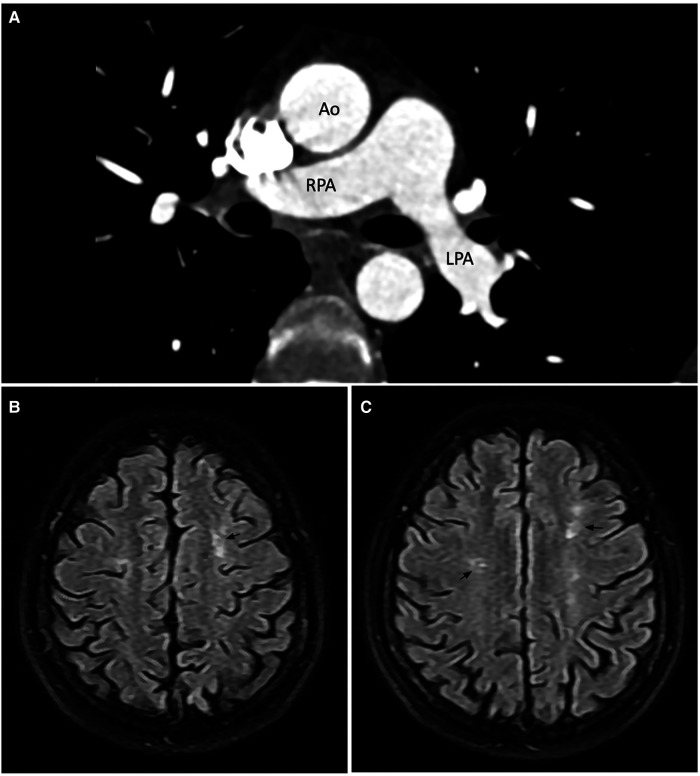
Computed tomography pulmonary angiogram (A) and brain magnetic resonance imaging scan (B,C). (A) No large pulmonary emboli on CTPA. (B,C) Axial FLAIR image showed bilateral hyperintense white matter lesions in the frontal lobes. The number of lesions is more than expected for the patient's age. Annotations: Ao, aorta; RPA, right pulmonary artery; LPA, left pulmonary artery.
